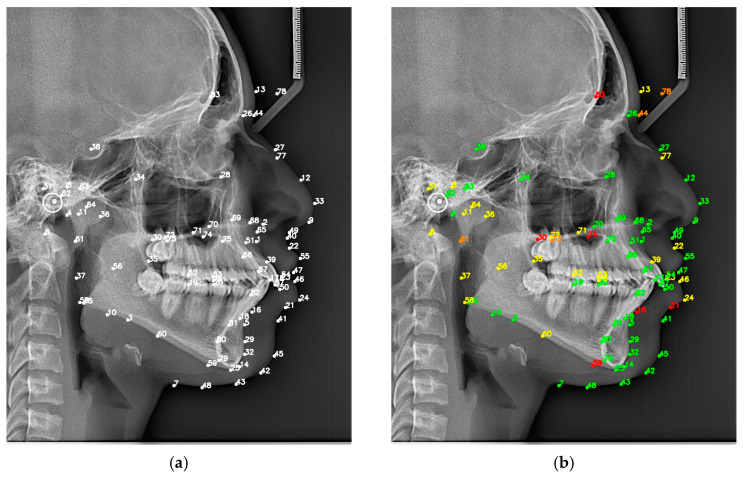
X-ray images with annotated cephalometric landmarks: (a) X-ray photos with manually marked cephalometric points; (b) X-ray photos with cephalometric points marked by algorithm.
